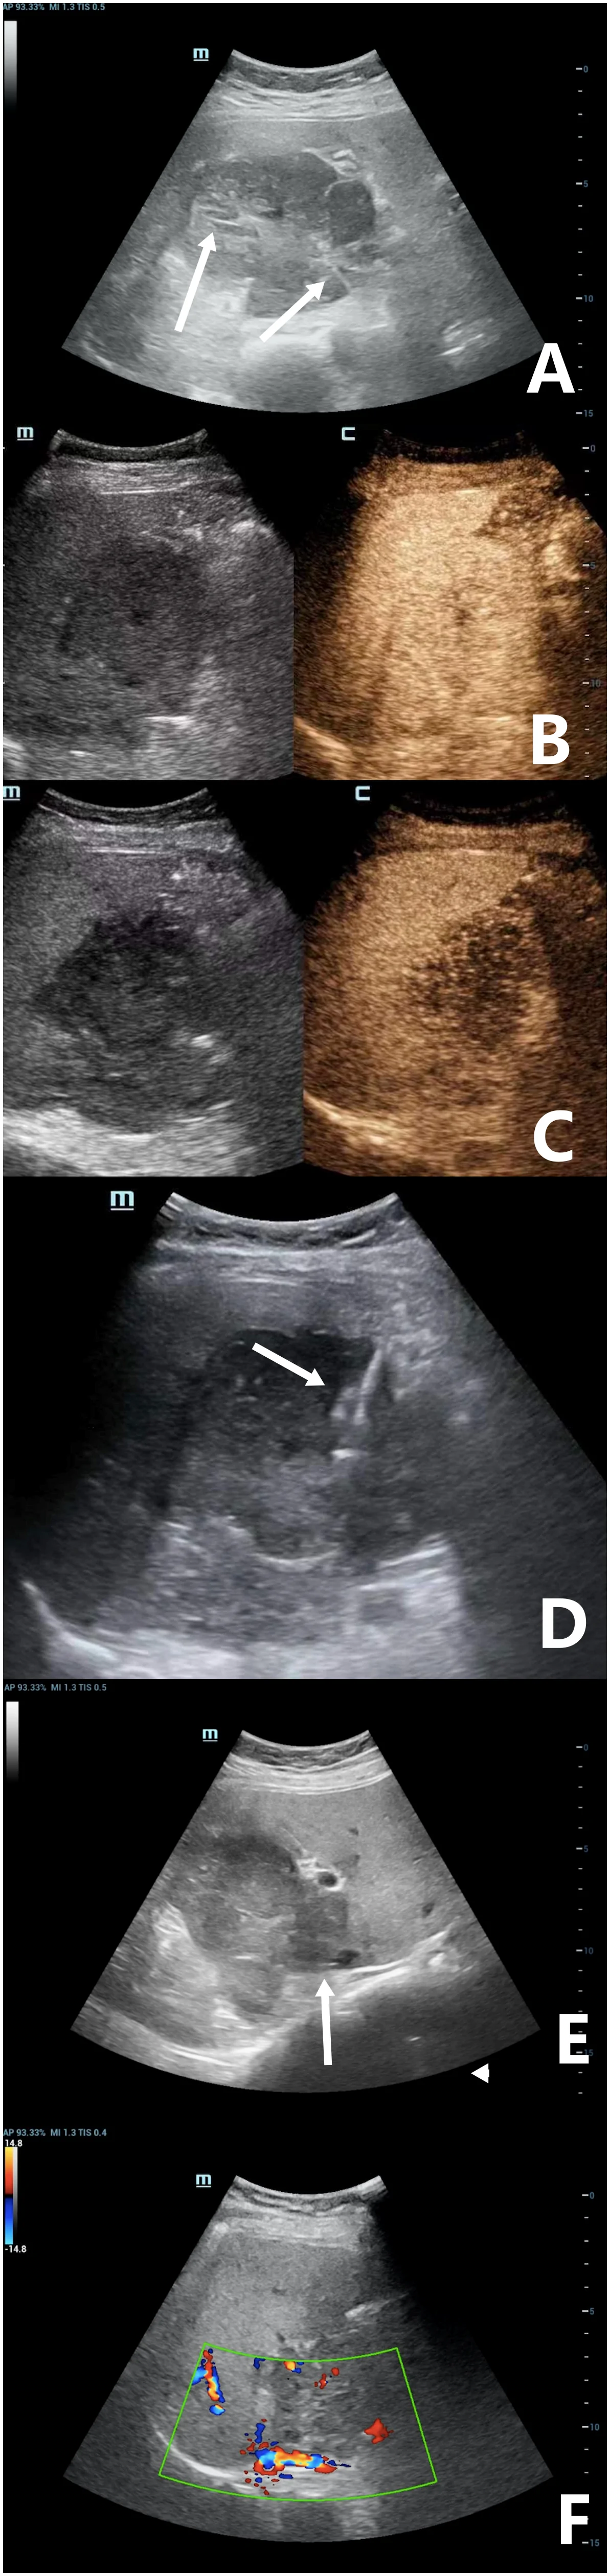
Ultrasound findings of IVC leiomyosarcoma. (A) Grayscale image shows a heterogeneous hypoechoic mass with multiple strip-like hyperechoic regions (arrows) and irregular anechoic areas suggesting necrosis. (B) Contrast-enhanced ultrasound (CEUS) in arterial phase demonstrates marked peripheral-to-center hyperenhancement. (C) CEUS in delayed phase shows progressive washout with persistent hypoechogenicity. (D) Ultrasound-guided percutaneous biopsy (arrow) using a 16G needle. (E) Retrospective cine-loop review reveals a hypoechoic component within the IVC lumen (arrow), continuous with the hepatic mass, initially overlooked. (F) Color Doppler with pulsed wave trace shows IVC luminal narrowing and increased flow velocity, initially misinterpreted as extrinsic compression rather than intraluminal tumor.
